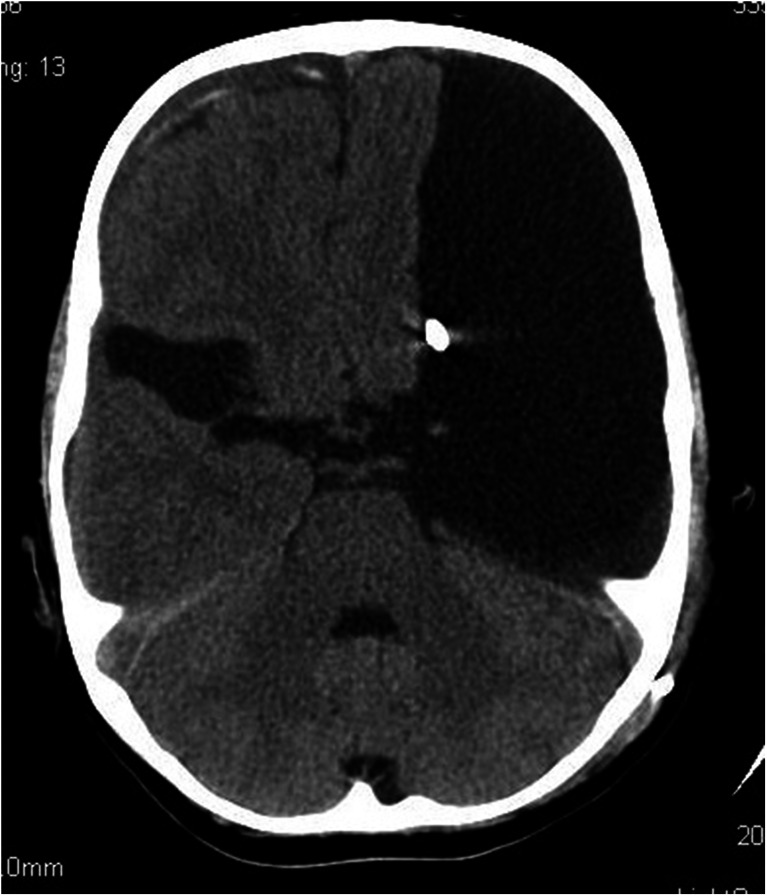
Status after implantation of a cysto-peritoneal shunt on the left side, a minimal mass effect, flat paracerebral hematoma hygromas in the vicinity of both frontal lobes, mild cerebral edema demonstrated as cerebral sulci, and fissure obliteration. Nonenhanced CT scan
